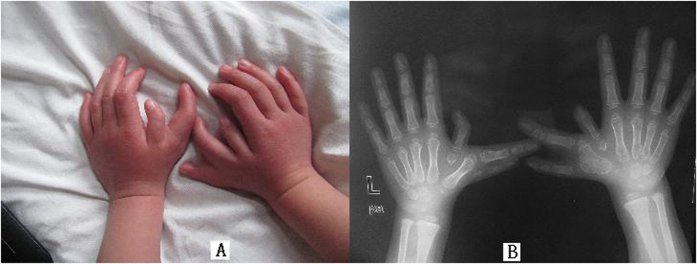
A view of both hands with 7 fingers of the five-year-old female proband (A Image of optical photograph; B Image of preoperative X-ray showed each hand has 2 triphalangeal thumbs).
